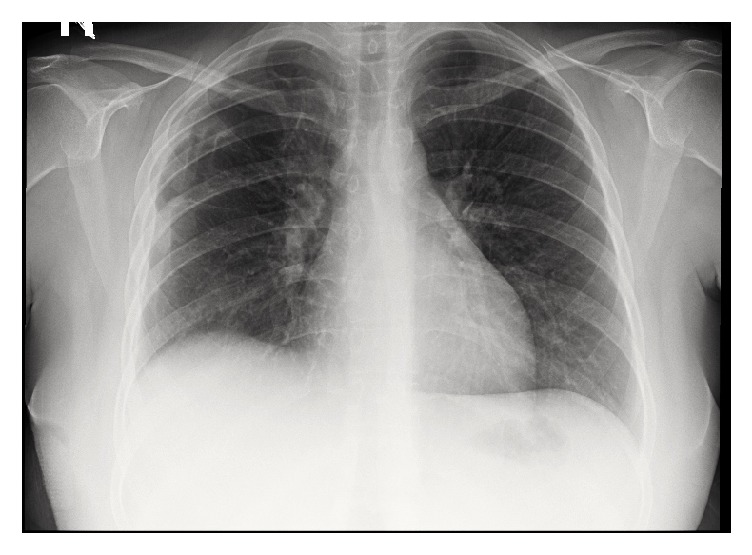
Chest X-ray on discharge showing resolution of the bilateral lung infiltrates, pleural effusions, and surgical emphysema.
